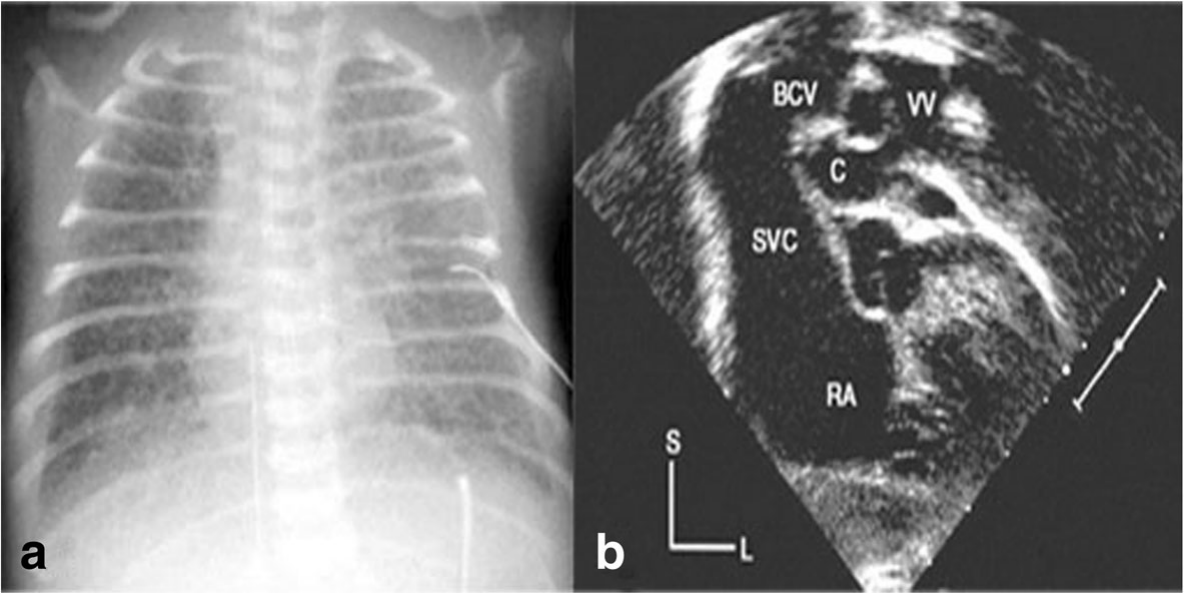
4-days old male with total anomalous pulmonary venous connection accompanied by pulmonary venous obstruction: a- CXR: Note mild cardiac enlargement and evidence of pulmonary venous hypertension (“ground glass” appearance). b- 2D-Echocardiography shows compressed vertical vein between lt.pulmonary artery and lt.main.bronchus
